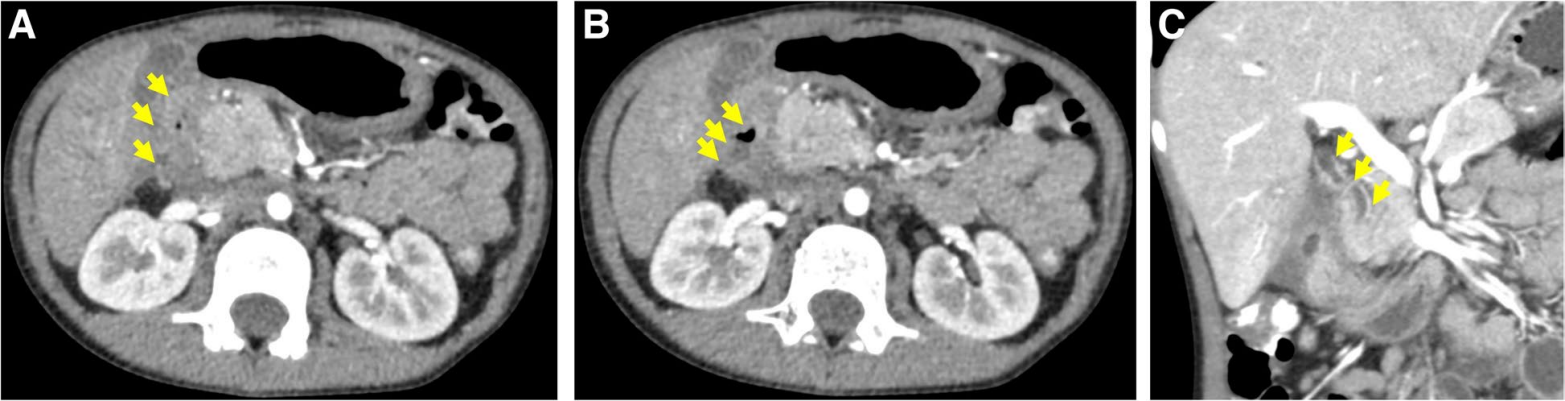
A Abdominal CT showing edematous duodenal wall thickening. B CT showing air-fluid levels on the right side of the duodenum. C The wall of the common bile duct is thickened with enhancement
